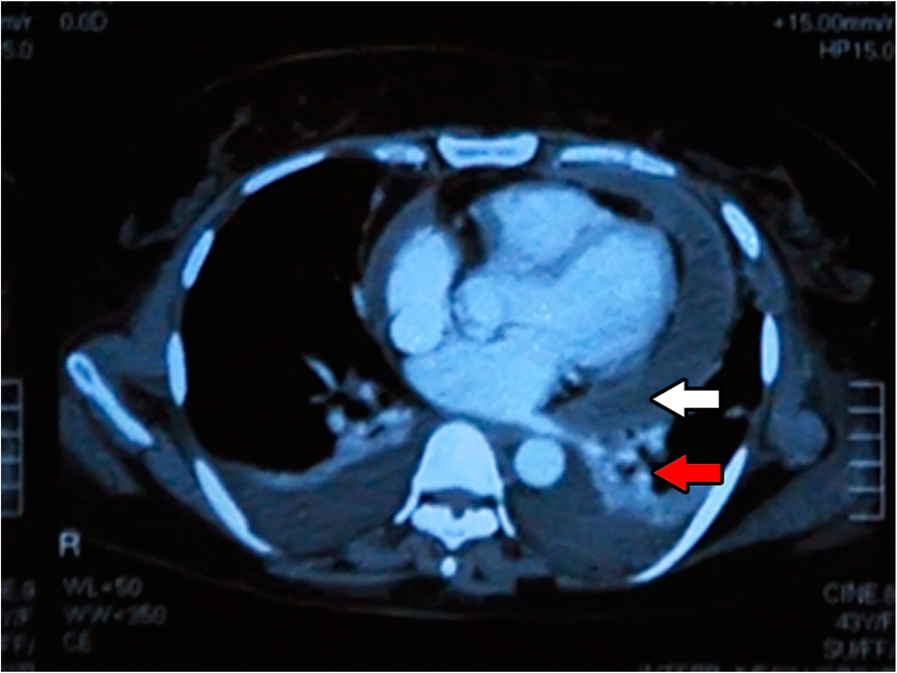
Contrast-enhanced CT of chest showing pericardial effusion (white arrow), bilateral pleural effusions and left-sided lung consolidation (red arrow).
